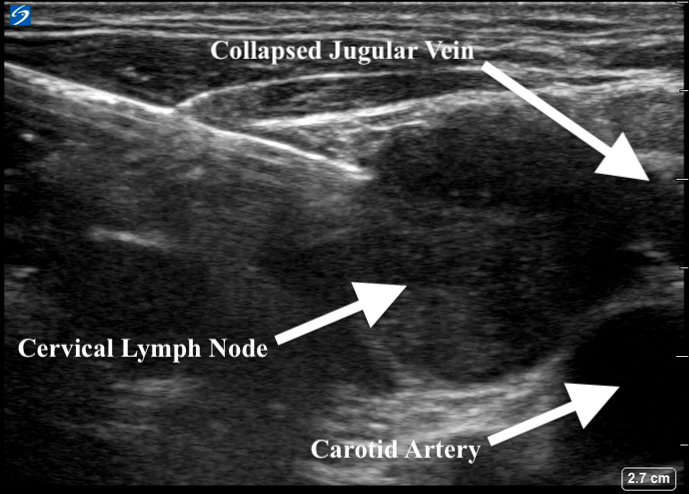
Soft tissue ultrasound image showing an the enlarged right supraclavicular lymph node withthe needle visible in its entire length.
